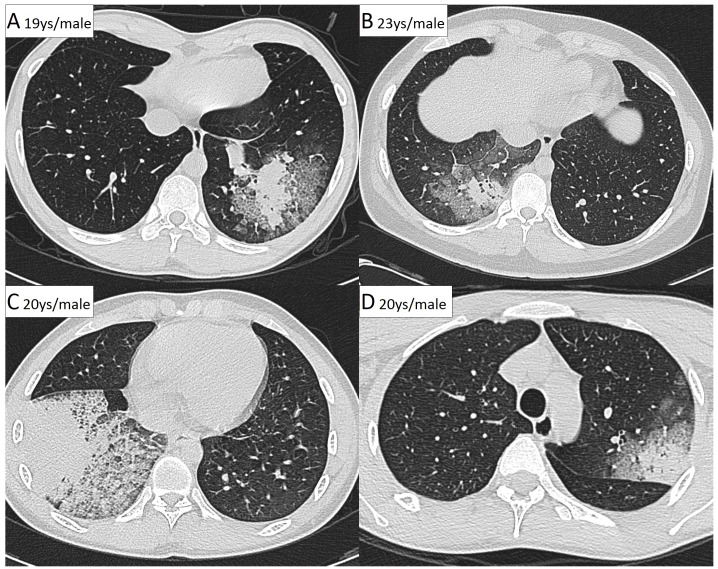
(A–D) Computed tomography scans of four patients with adenoviral pneumonia. Parenchymal opacities (consolidation and ground-grass opacity) with septal thickness were distributed along bronchovascular bundles or in the subpleural lung.
